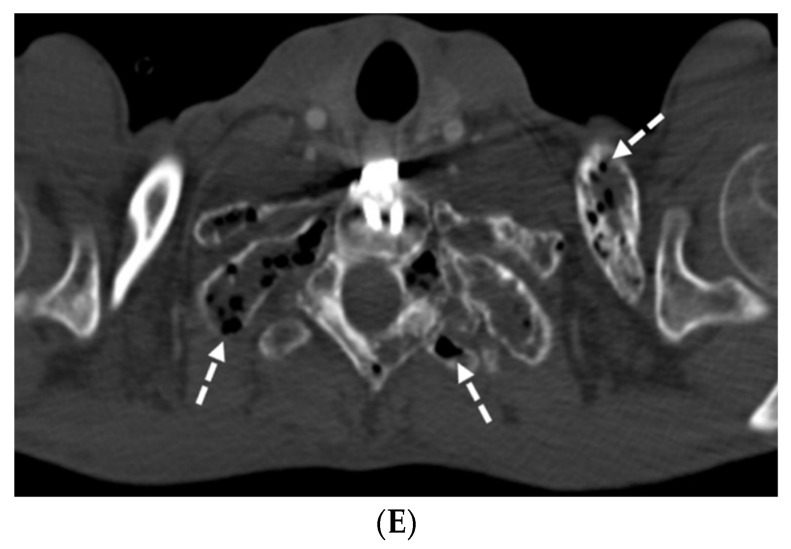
A 29-year-old male with diffuse pulmonary lymphangiomatosis presents with dyspnea. A frontal chest radiograph (A) and axial chest CT images (B–E) demonstrate smooth interlobular septal thickening (solid white arrow), multiloculated pleural effusions (stars on chest radiograph), diffuse infiltration of mediastinal and extrapleural fat (open white arrows), and lytic bone lesions (dashed white arrows). Multiple hyperattenuating punctate foci within pleural spaces represent residual lipiodol from prior lymphangiography (curved white arrow).
